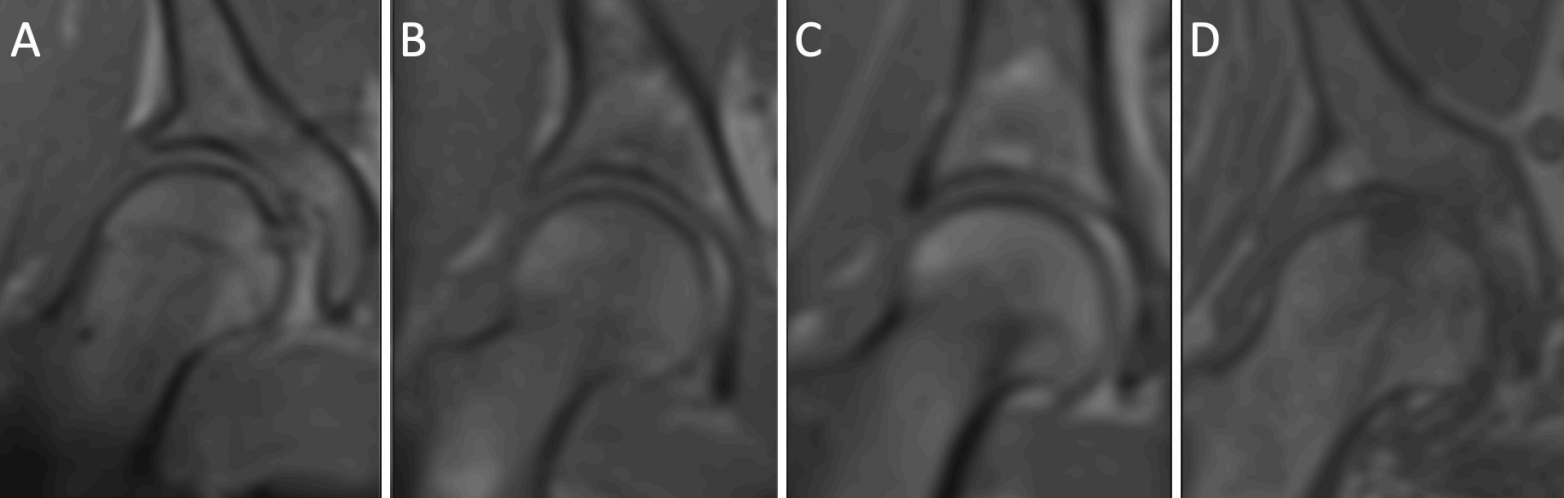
Examples of T1 vibe dixon in phase of the degenerative states of the OA MRI score. A: Grade 0 –no degeneration; B: Grade 1 –Slight narrowing of the medial joint gap and small osteophytic lipping; C: Grade 2 –narrowing of the medial joint gap and increased osteophytic lipping; D: Grade 3 –loss of the medial joint gap, osteophytic lipping as well as pseudocystic lesions.
